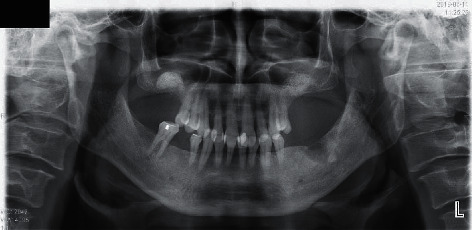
No maxillary sinusitis and the graft material of bilateral maxillary sinus floor was detected in postoperative panoramic radiography.
